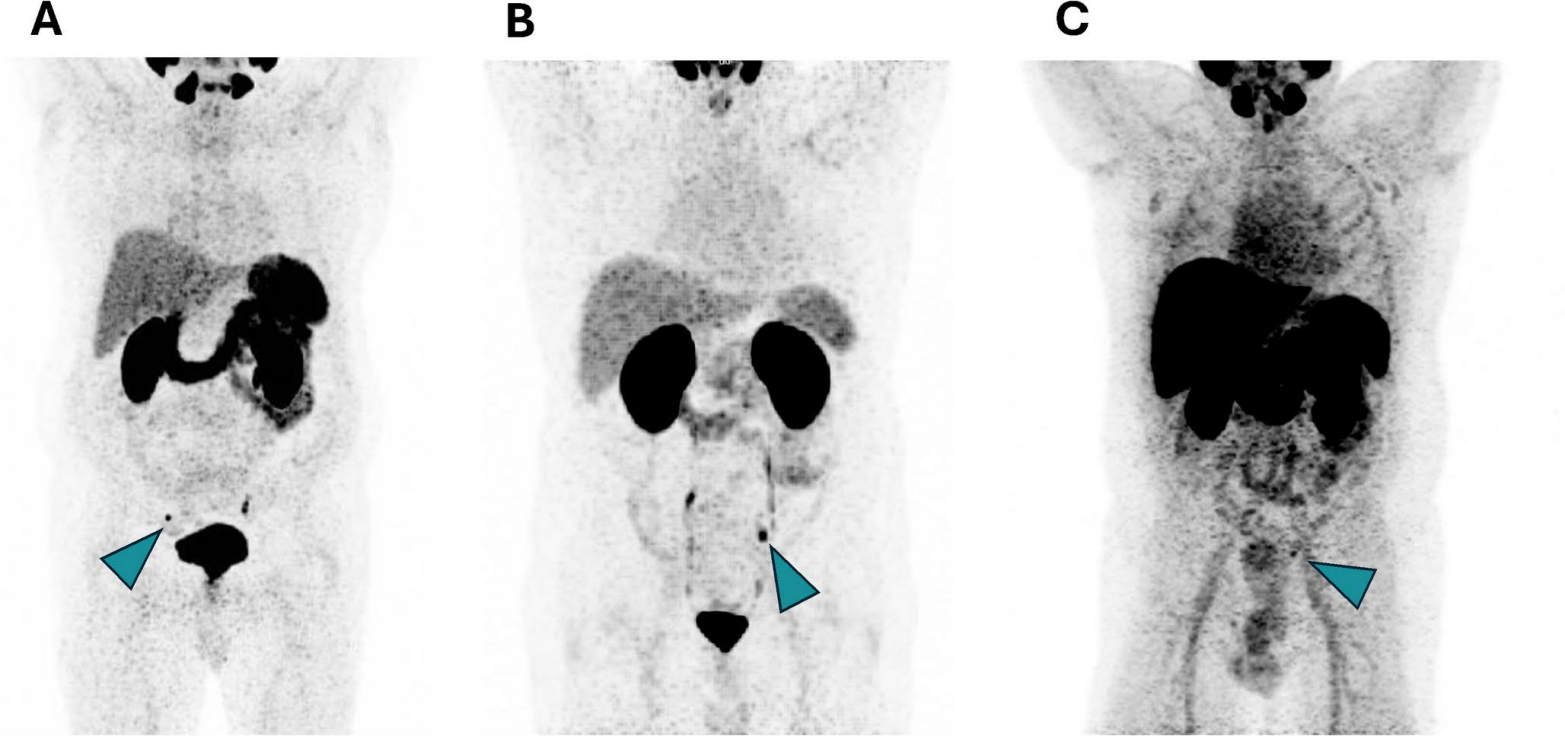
Maximum intensity projections of positive PSMA PET/CT scans with successful localization of BCR (lymph node metastasis) using the three different radiotracers A) [^68^Ga]Ga-PSMA-11, B) [^68^Ga]Ga-PSMA I&T and C) [^18^F]PSMA-1007. Blue arrows indicate the localization of BCR
